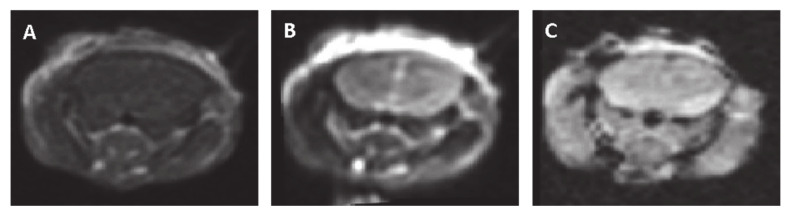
Representative MRI slices obtained 48 h post-pulsed electrical fields (PEFs) application (100 pulses at 300 V) (A). Contrast-enhanced T1-MRI. (B) T2-MRI. (C) Gradient echo (GE) MRI.
